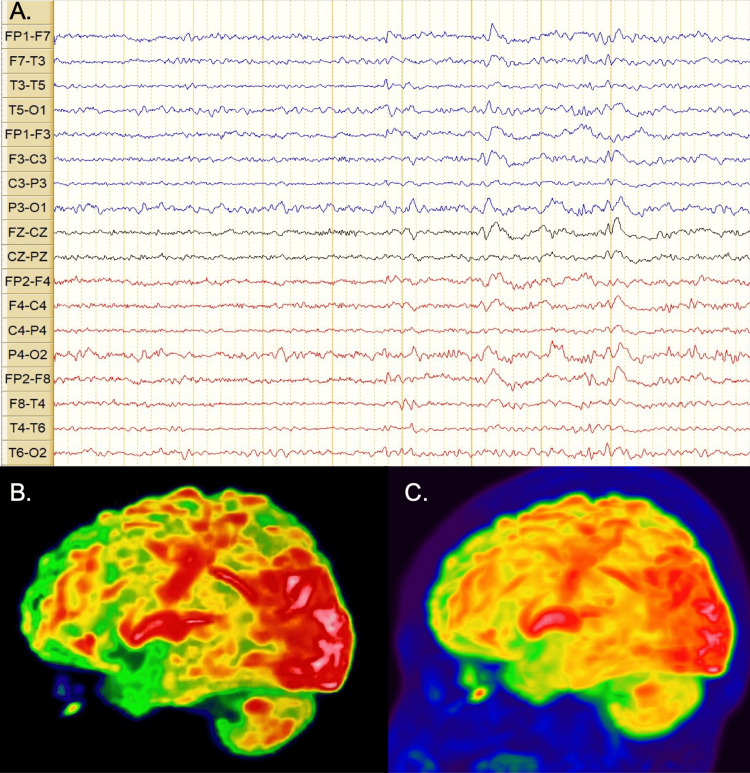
Follow-up cognitive, electroencephalographic, and metabolic assessment after clinical recovery (A) Follow-up EEG obtained during outpatient evaluation showing bilateral frontotemporal focal epileptiform activity, with left-sided predominance. (B) Follow-up brain 18F-FDG PET/CT at one month demonstrating persistent but decreased cortical hypermetabolism (residual high-uptake cortex, red on the color scale, reduced in extent compared with the baseline study in Figure 1B). (C) Follow-up brain 18F-FDG PET/CT at eight months showing further metabolic improvement, with marked reduction of the red (high-uptake) cortical areas EEG: electroencephalography; 18F-FDG PET/CT: 18F-fluorodeoxyglucose positron emission tomography/computed tomography
